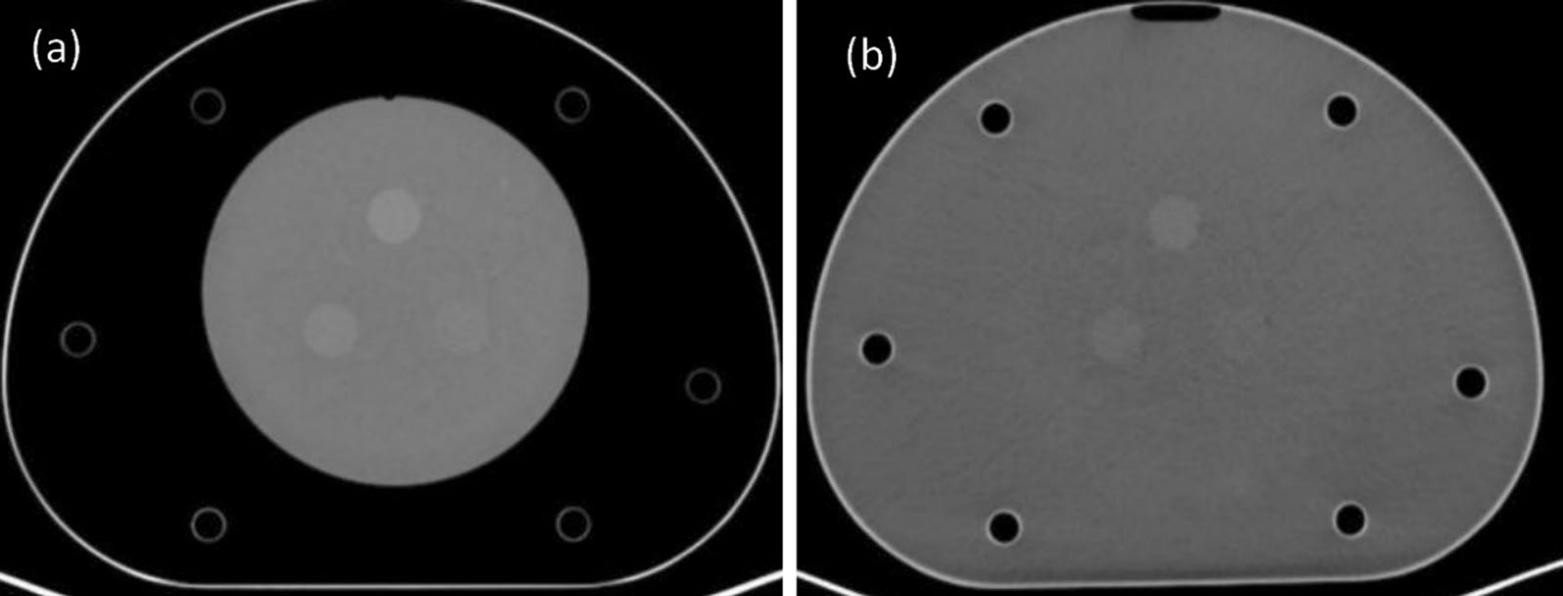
The verification of water-equivalent characteristic in the background of low-contrast CT module for injection of purified water. (a) Before, (b) after
